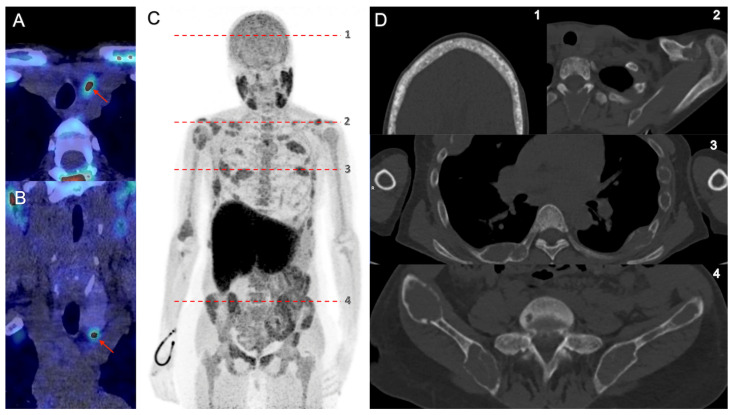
A 27-year-old woman with a history of kidney transplantation and hemodialysis was referred for sHPT. 18F-FCholine PET/CT revealed a left inferior hyperfunctioning parathyroid gland (arrows, (A): axial PET/CT, (B): coronal PET/CT) and multiples bones uptakes ((C): MIP PET anterior image) corresponding to both cystic lesions and patches of osteolysis (D) of the distal clavicles (D.2), a “salt and pepper” appearance of the skull (D.1), and both bone cysts and brown tumors in axial and extra-axial bones (D.3–4) allowing the diagnosis of osteitis fibrosa cystica (OFC). The patient was operated on, and pathology revealed a left inferior parathyroid hyperplasia.
